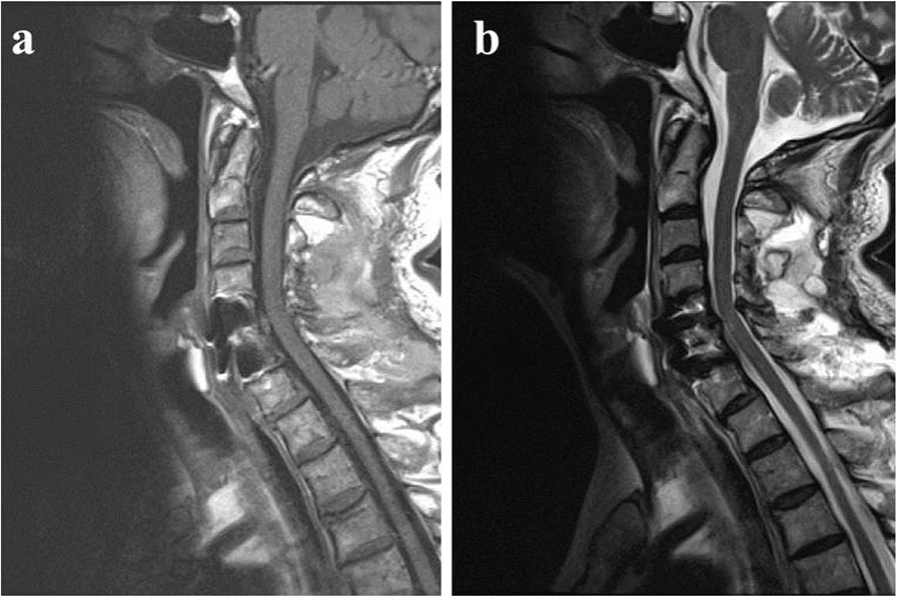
Magnetic resonance imaging three months after surgery. Space in the spinal canal has increased, and swelling of the spinal cord has decreased three months after surgery. (a) T1-weighted image; (b) T2-weighted image.
